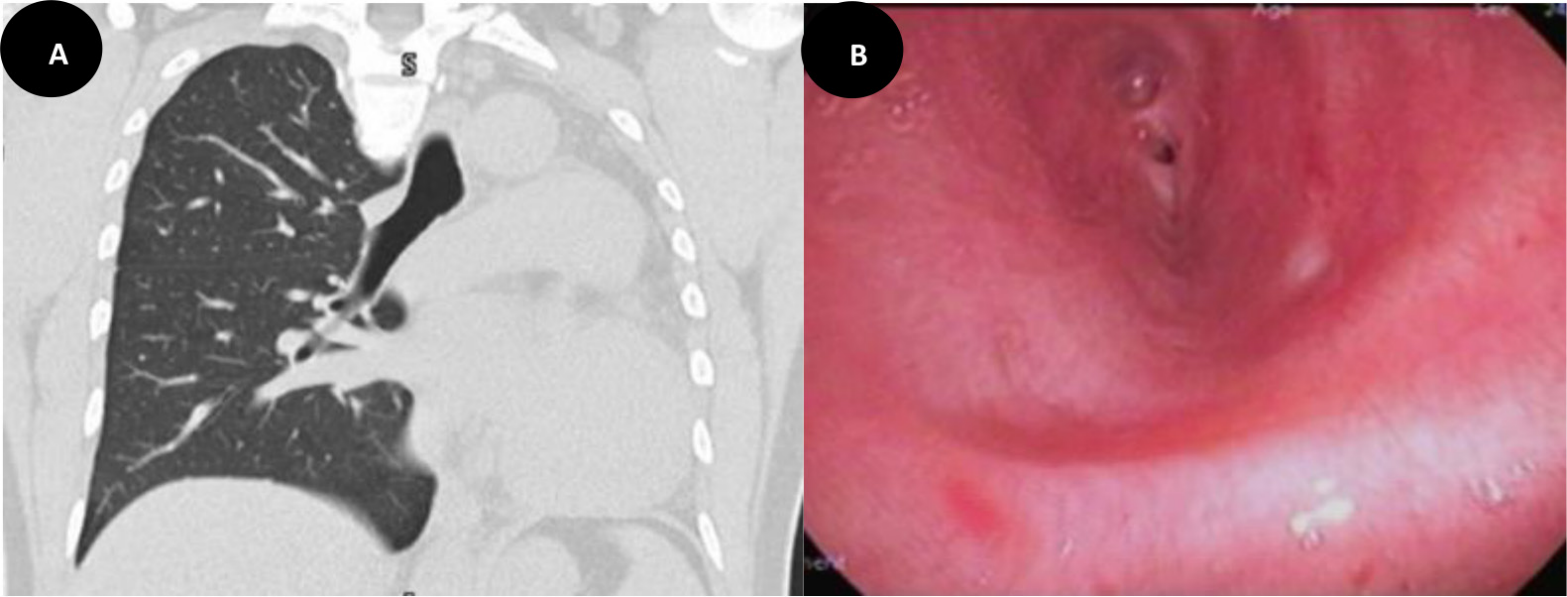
A coronal CT chest view shows a complete absence of left lung with a left side mediastinum shift and hyperinflation of the right lung (A). A bronchoscopy view demonstrates a rudimentary left main bronchus with complete absence of the remaining left bronchial tree (B).
